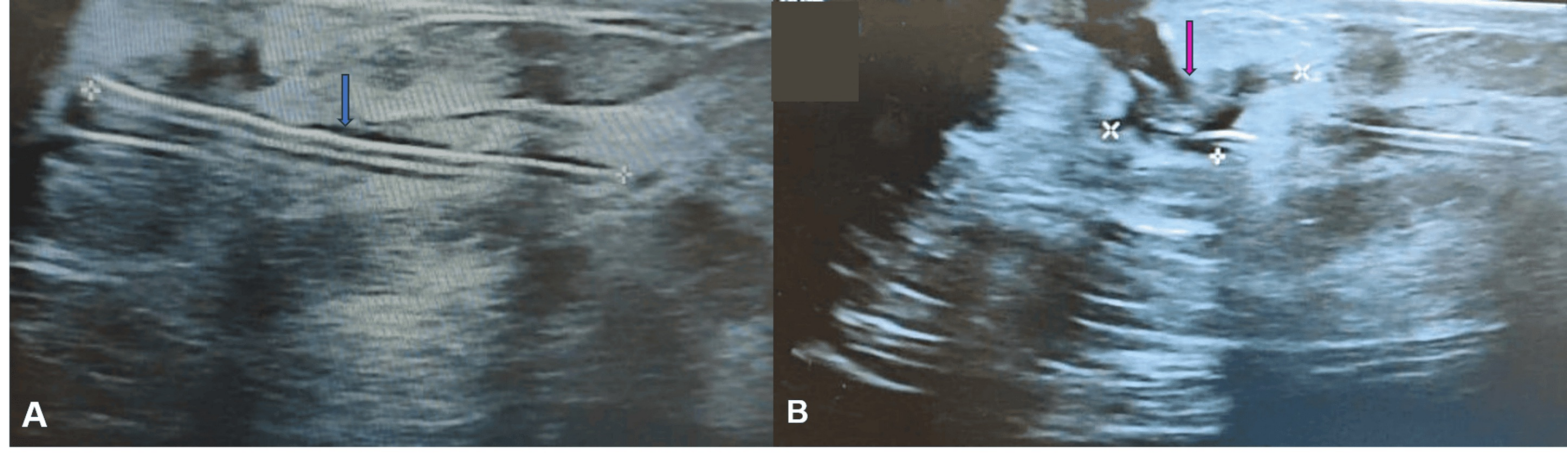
Ultrasonography images of the left breast (case one) (A) A linear hyperdense structure with central linear hypodensity consistent with a needle is seen (blue arrow). (B) An irregular hypodense area near the linear hyperdense needle with a tract-like extension into the skin surface is indicative of a collection (pink arrow).
